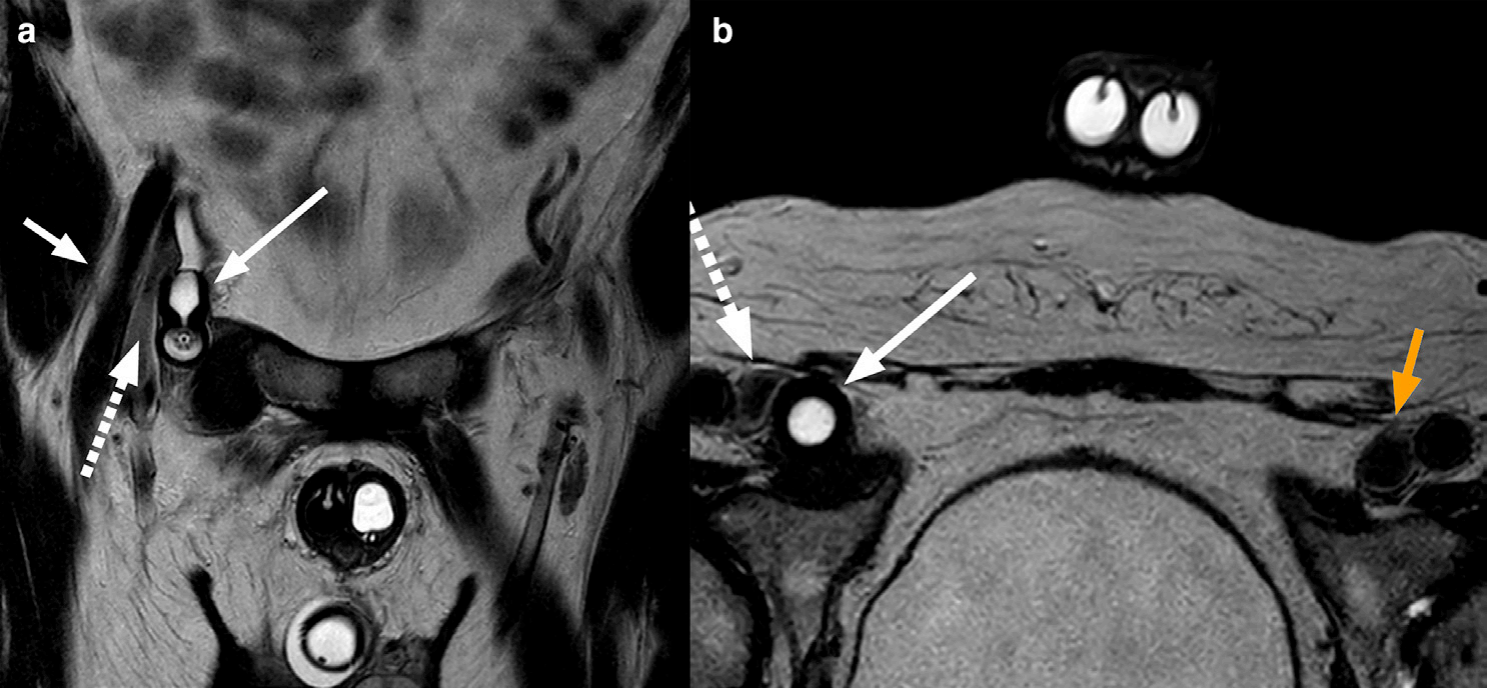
MRI T2 TSE in coronal (a) and transverse (b) views show the neck of the reservoir (arrow) extending into the femoral canal with compression of the right common femoral vein (dashed arrow), compared with the contralateral side (orange arrow, b). The femoral nerve lies laterally (short arrow, a) and is not compressed by the reservoir. A cross-section of the inflatable penile implant and scrotal pump are also visible. TSE, turbo spin echo.
